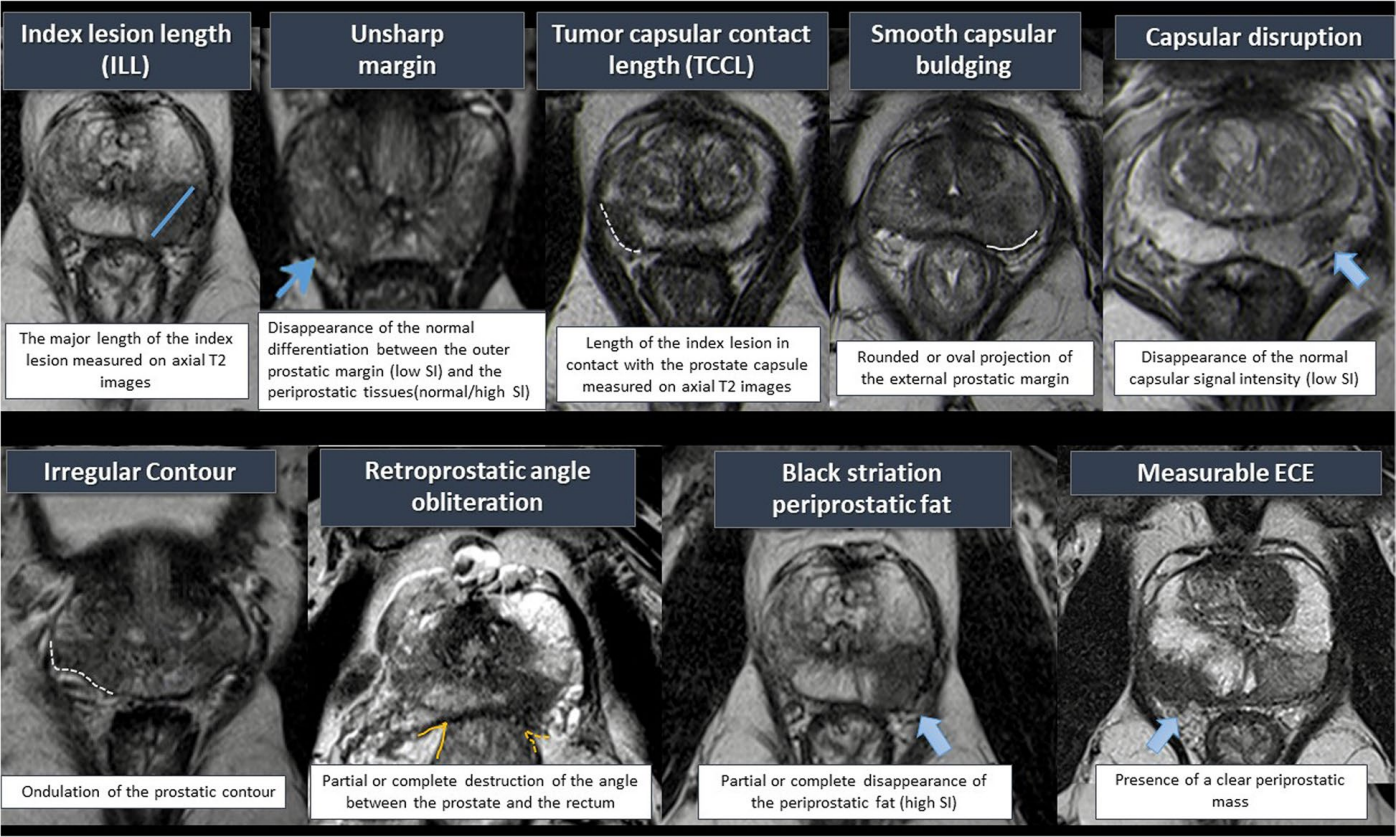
MRI Semantic features for detection of pECE
